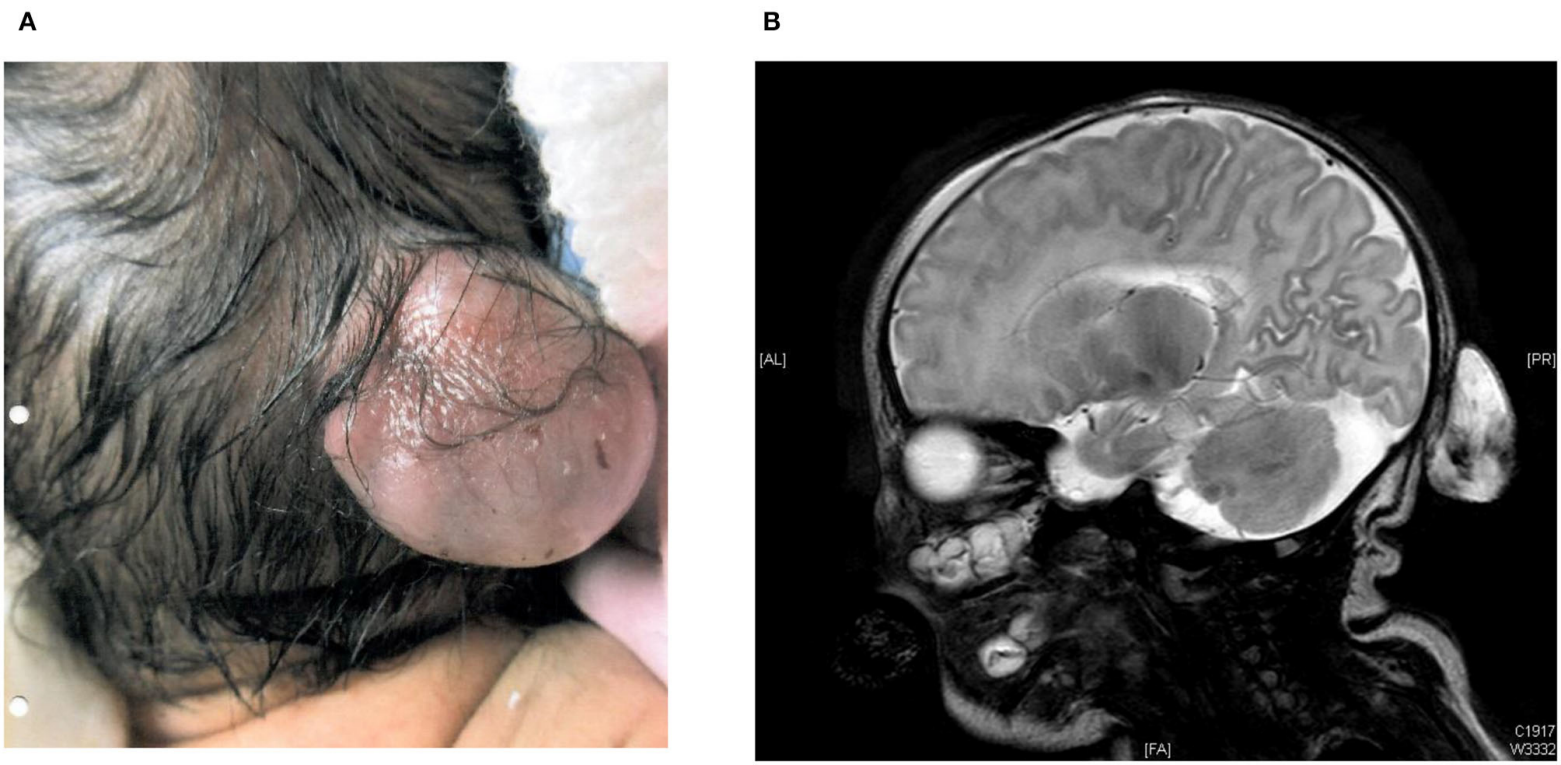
(A) 30 mm × 20 mm × 30 mm encephalocele in occipital region at birth in the younger brother. (B) MRI brain on day 2 of life from the younger brother.
